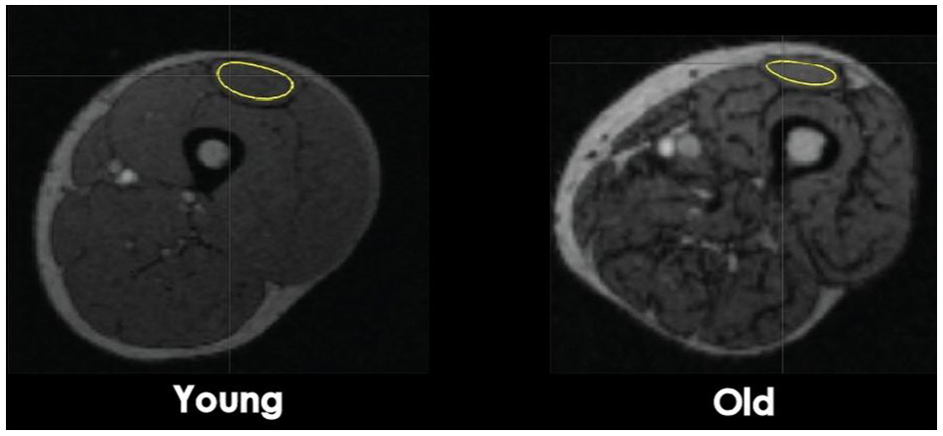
Transaxial CT images at a mid-thigh level. Computed Tomography images of a cross-section of the femoral region from a young and old man subject. The regions of interest (ROI) used for glucose uptake heterogeneity analysis were drawn around the quadriceps femoris (QF) muscles, four knee flexors muscles, and three hip muscles. For muscle volume analysis, ROIs were draw around the four next extensor muscles. ROI of the rectus femoris is shown as an example.
